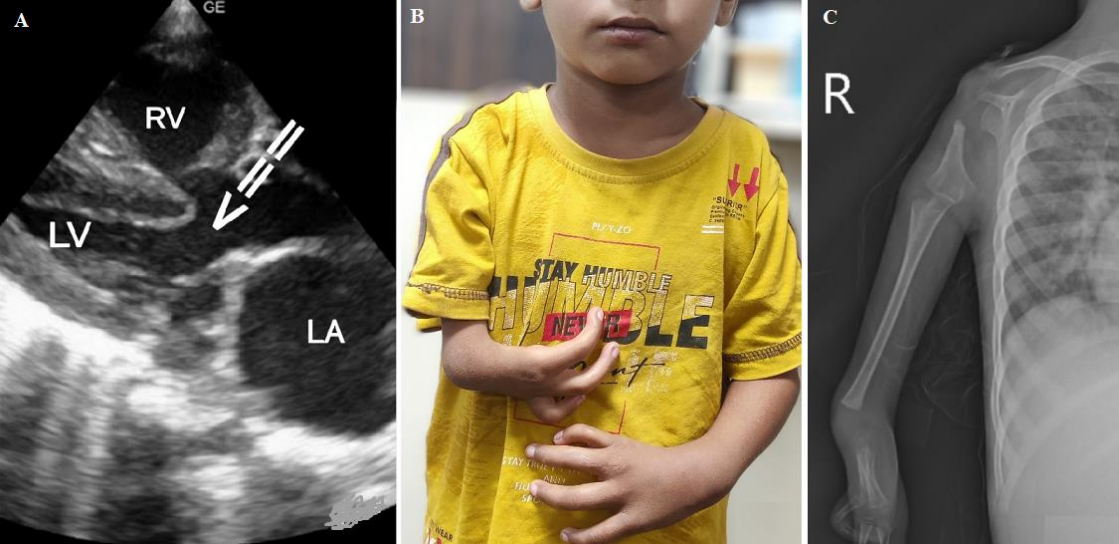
A) 2-D echo of the patient showing ventricular septal defect (VSD) (LA: left atrium; RV: right ventricle; LV: left ventricle); B) clinical photo of the patient with upper limb skeletal deformity; C) X-ray of right upper limb showing hypoplastic humerus with radial and ulnar bone defect
